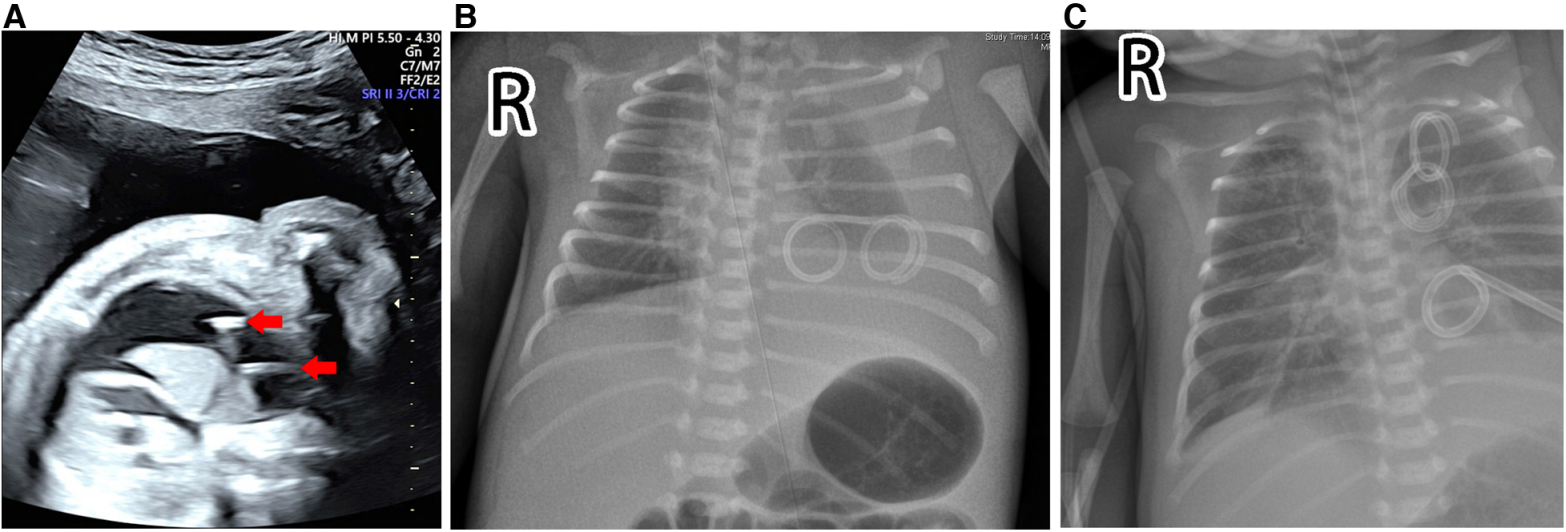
Prenatal ultrasound screening and postnatal chest radiography. (A) The ultrasound examination identified reaccumulated pleural effusion and clearly visualized the entire double-pigtail shunt (red arrows) located within the fetal chest, while the intra-amniotic segment remained invisible. (B) The chest x-ray displayed a massive left pleural effusion with dislodgement of the thoracoamniotic shunt. (C) The chest x-ray showed that the dislodged shunt had migrated to the left upper thorax.
